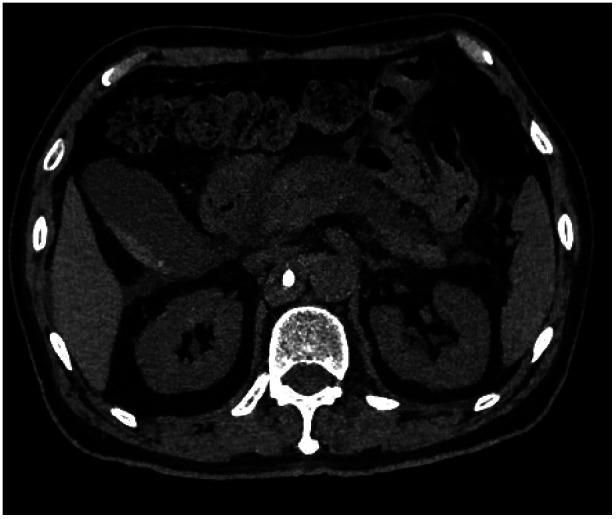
Abdominal CT showing obvious peripancreatic effusion with fuzzy edges, and high density bone cement shadow was seen in the right anterior of L1 vertebra.
